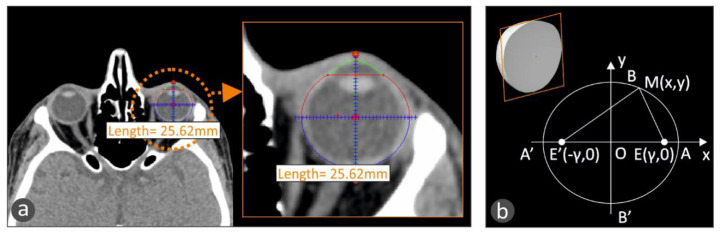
(a) Fitting an ellipse in the human eye circumference to more accurately capture the eye geometry in a CT scan image with the developed methodology. (b) The ellipse geometry that the human eye follows, according to human anatomy [16].
